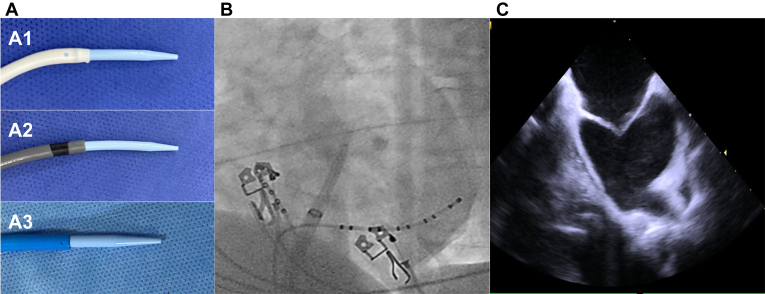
Confirming the position of the sheath. A: Sheaths with the wire at the tip of the dilator: nondeflectable sheath (A1), deflectable sheath (A2), cryoballoon sheath (A3). B: Fluoroscopy view perpendicular to the mitral valve with the sheath parallel to it. C: Intracardiac echocardiography showing the system being pushed toward the atrial septum, with satisfactory distension of the fossa ovalis.
